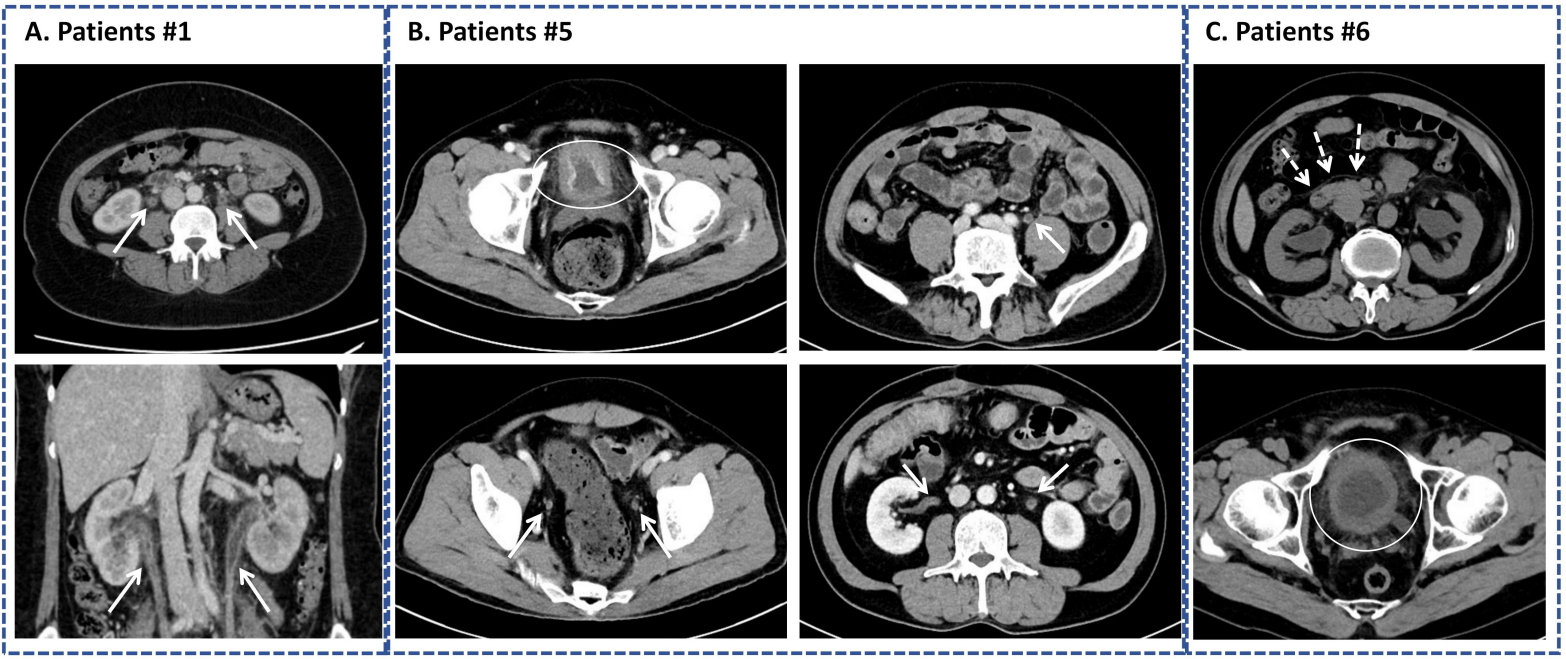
Characteristic computed tomography (CT) manifestation of immune checkpoint inhibitors (ICIs)-related ureteritis and cystitis. (A) Patients No. 1. (B) Patient No.5 with mild urinary irritative symptoms. (C) Patient No.6 with visualization of the anterior renal fascia.Thickening of bladder wall is outlined by a white oval. (⇢) represents anterior renal fascia. (→) represents thickening or enhancement of the ureteral wall and dilation of the renal pelvis and ureter.
